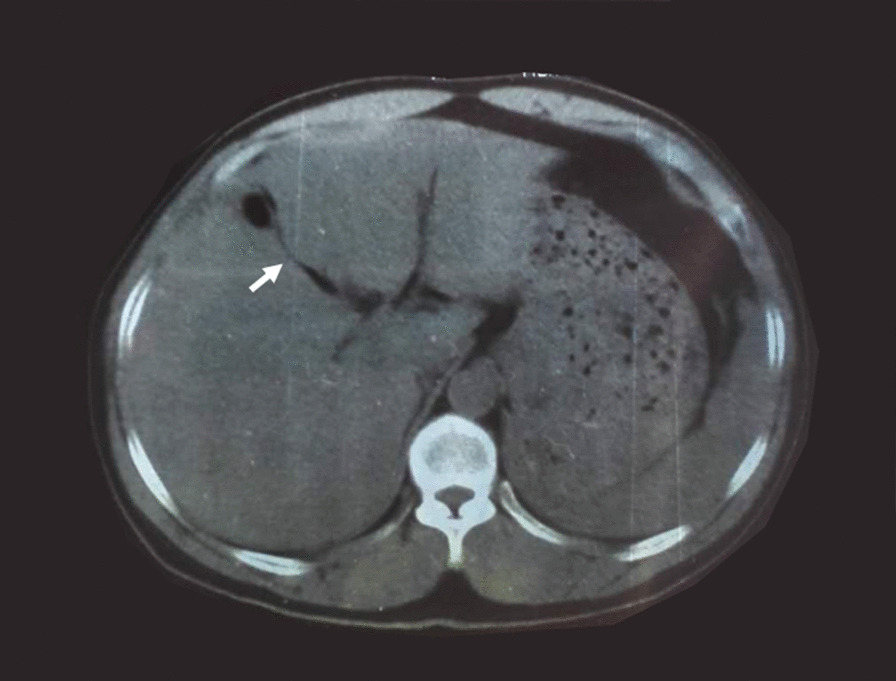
Abdominal computed tomography image showing liver lacerations (arrow)
